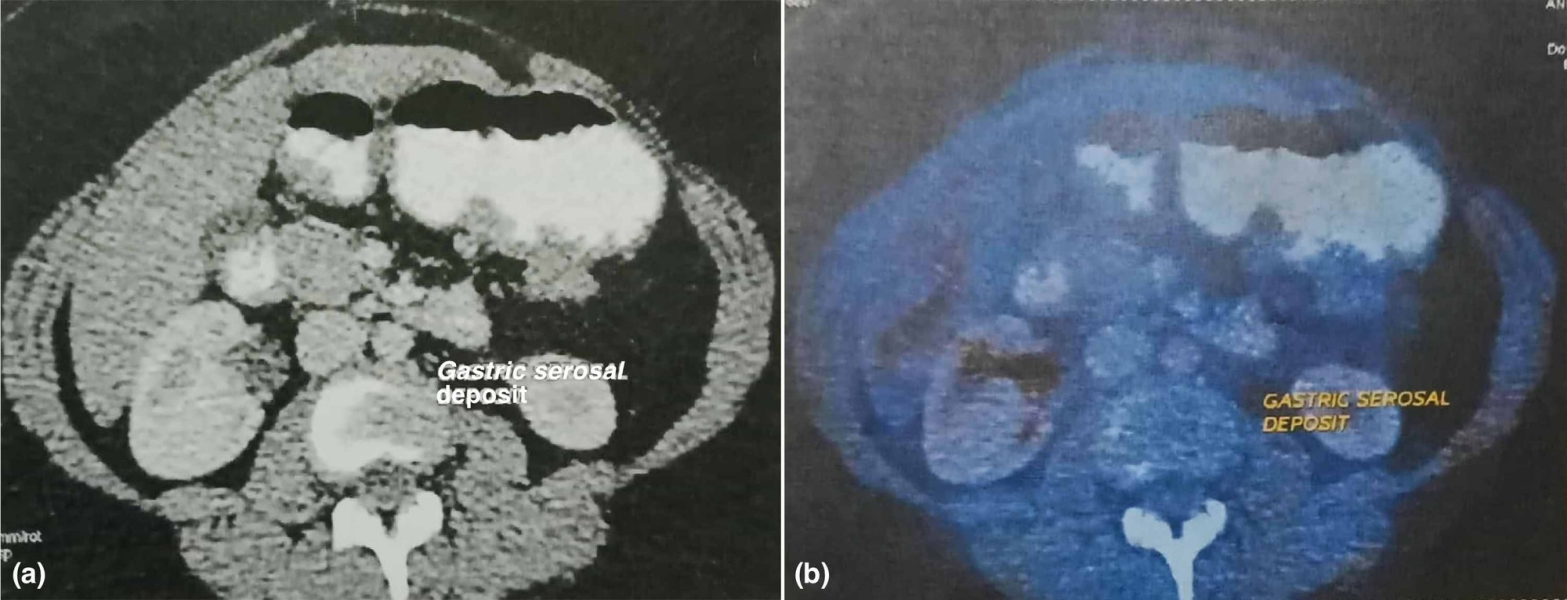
(a) Contrast-enhanced CT abdomen image shows gastric serosal deposits. (b) Corresponding fused PET-CT image shows gastric serosal deposits. A peritoneal nodule of size 25 x 20 mm is noted abutting the greater curvature of the stomach with mild metabolic activity. PET-CT, positron emission tomography-computed tomography.
